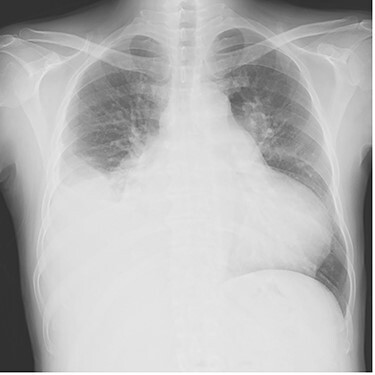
Plain chest radiograph at the time of admission.
